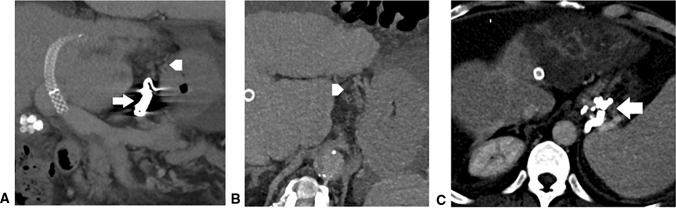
a–c: (a) CT after coil embolization of coronal vein (arrow) with distal not closed varix vessels (arrowhead) (cor. MPR); (b) distally not closed varix vessels (arrowhead) (ax); (c) CT after glue embolization of varix vessels forming “cast-like” formation (arrow)
